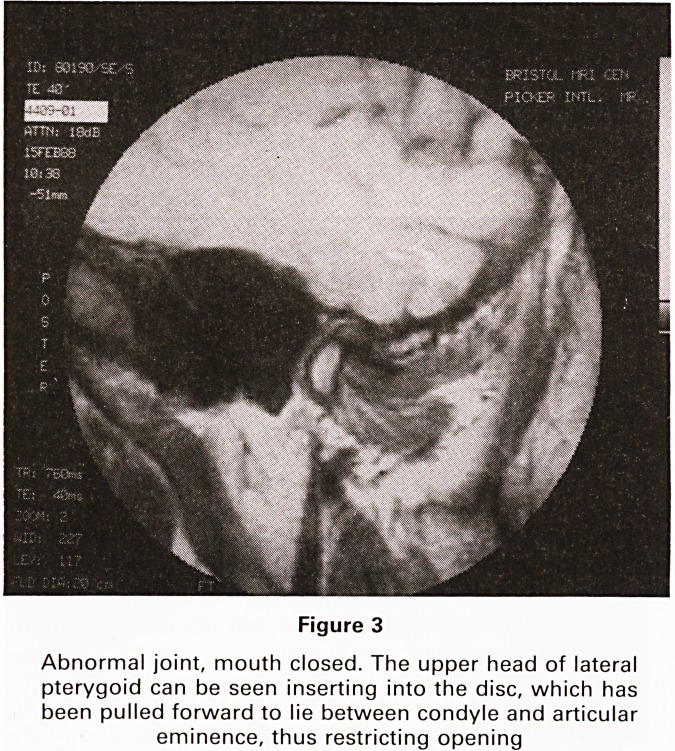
# Magnetic Resonance Imaging in the Investigation of Temporo-Mandibular Joint Disorders
